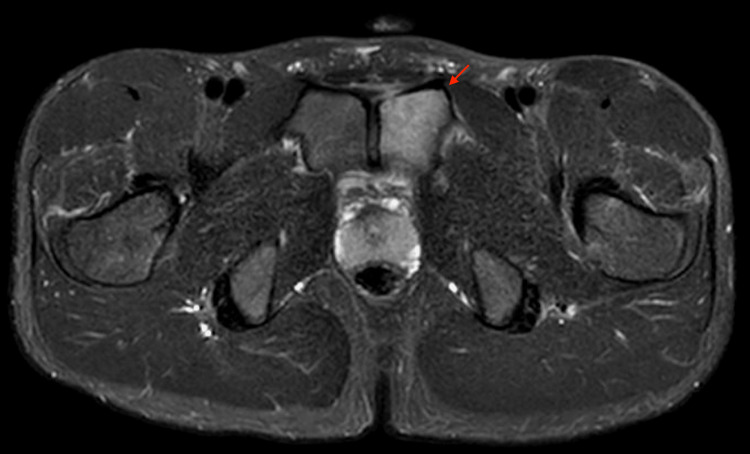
Pelvic magnetic resonance imaging on day 10 after onset. Coronal magnetic resonance imaging short-TI inversion recovery of the pubic region revealed a high signal in the left pubis, which was indicative of osteomyelitis.
